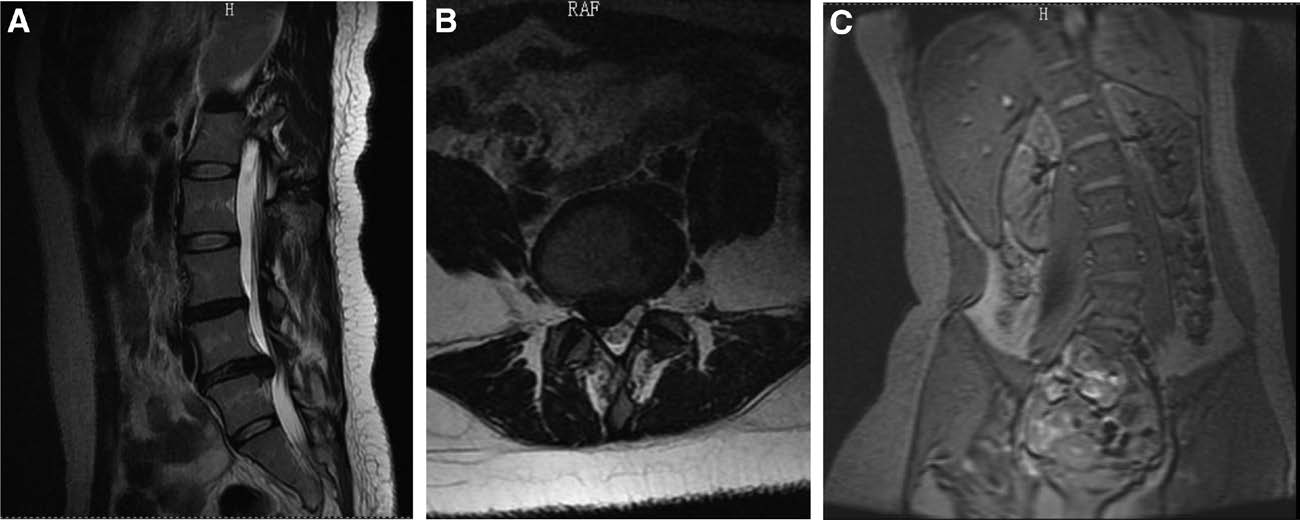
On May 4, 2017, the patient complained of recurrent right-sided lower extremity reflex pain with activity limitation and lumbar pain for more than 3 months. An MRI examination was performed at the local hospital, and the MRI scan of the lumbar spine suggested: L4/5 disc degeneration and bulging; L5/S1 disc right posterior prolapse with right nerve root compression. Mild degeneration of the lumbar spine, left lateral curvature, and lumbarization of the sacral spine with endplate inflammation at the superior margin of S1 considered (Fig. 1A–C).
